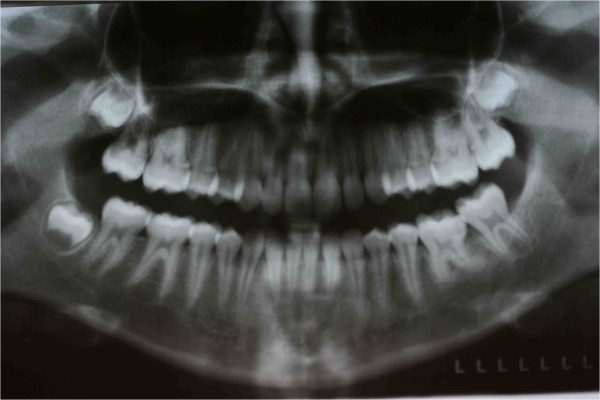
Post-treatment radiograph.
